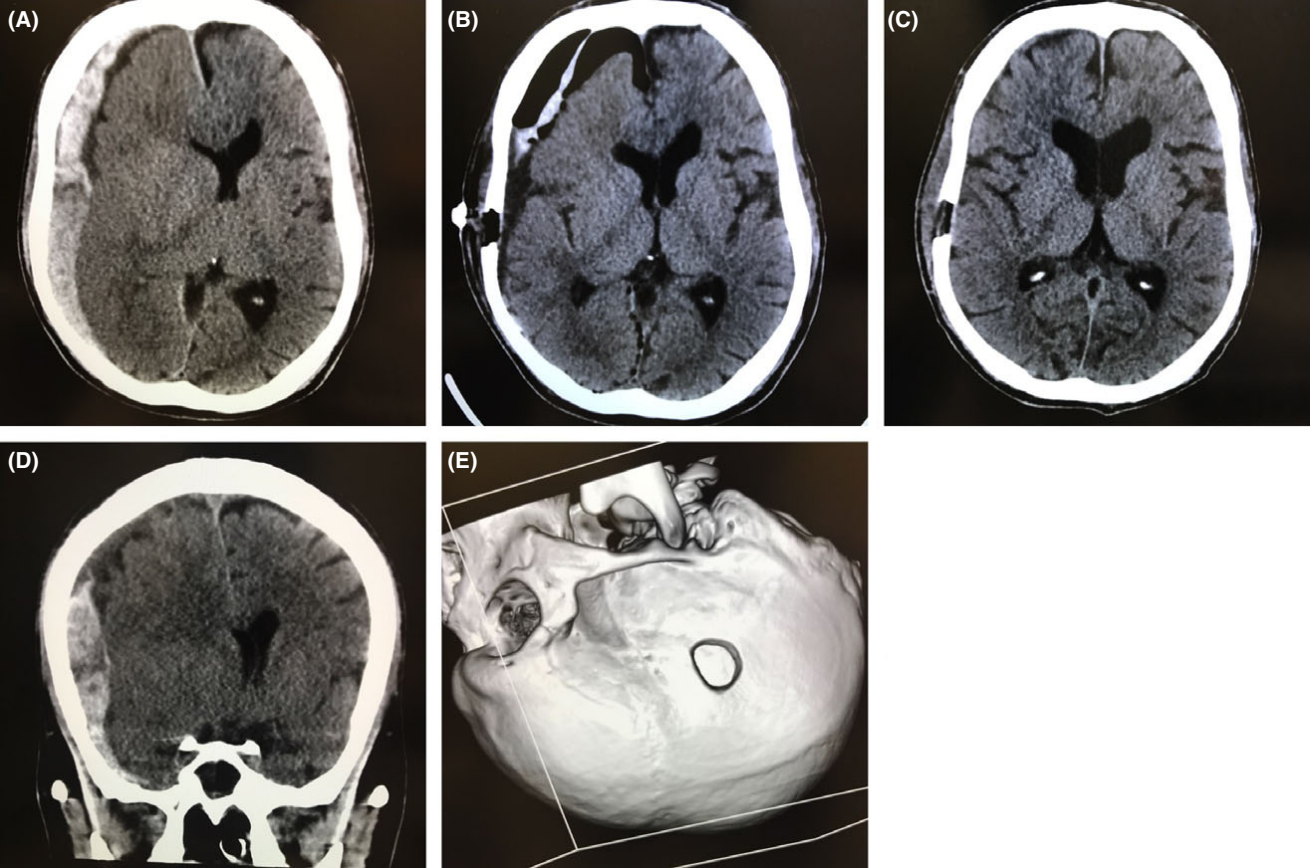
Preoperative and postoperative computed tomography scans in a 31‐year‐old man with acute subdural hematoma, with a history of schizophrenia and epilepsy. A, D, Massive hematoma observed preoperatively. B, C, One day (B) and 1 month (C) following endoscopic surgery, the mass effect was decreased. E, Surgical window for endoscopic procedure, showing the small hole (diameter, 20 mm) for inserting and maneuvering endoscopic instruments.
